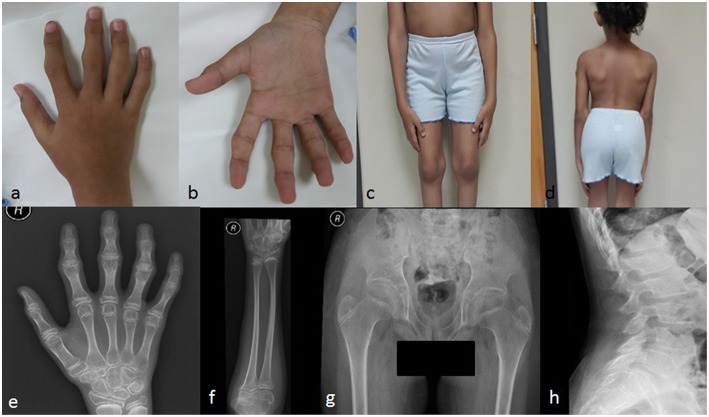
Clinical pictures of the patient IV-4 (a) showing camptodactyly of all fingers, with prominent PIP and DIP joints (b) represents the inability of the patient to make a fist (c) shows the prominent knee joints (d) shows short trunk. Radiological findings of the patient IV-4. (e) an X-rays of the right hand shows the irregular articular surface of the PIP joints with relative decrease in joint space and enlargement of the epi-metaphysis of the interphalangeal joints (f) shows elbow joints with prominent capitulum and trochlea of the distal humerus and irregularity of the articular surface (g) shows hips with bilateral fine irregularities of articular surfaces (h) shows the anterior beaking of the vertebral bodies with mild form of platyspondyly.
